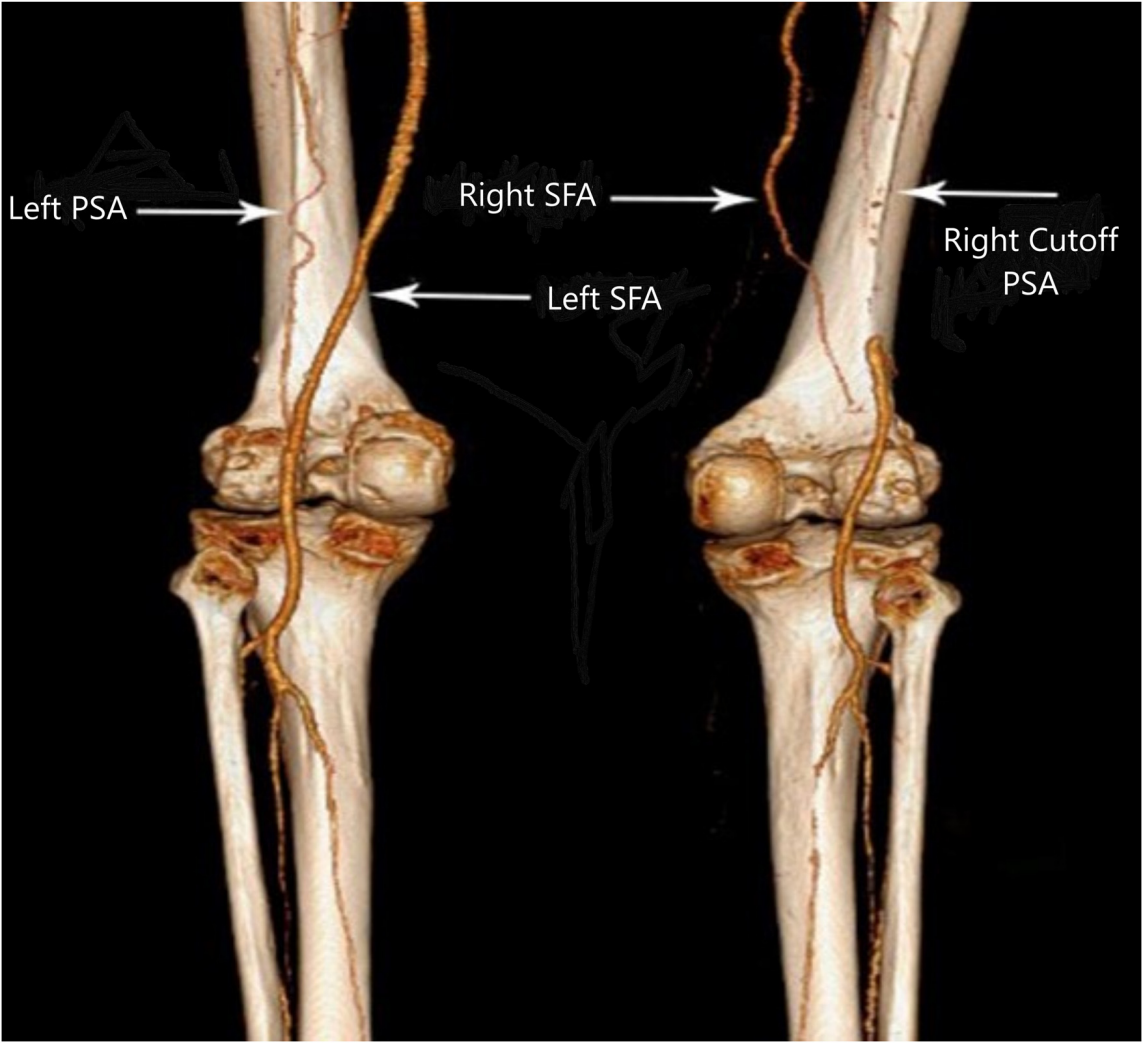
Posterior lateral view 3D CT reconstruction demonstrated right SFA have no continuity to popliteal artery but the left SFA have continuity to popliteal artery. The left PSA extending downward and connected to left popliteal artery. Also, the right PSA cutoff is seen.
